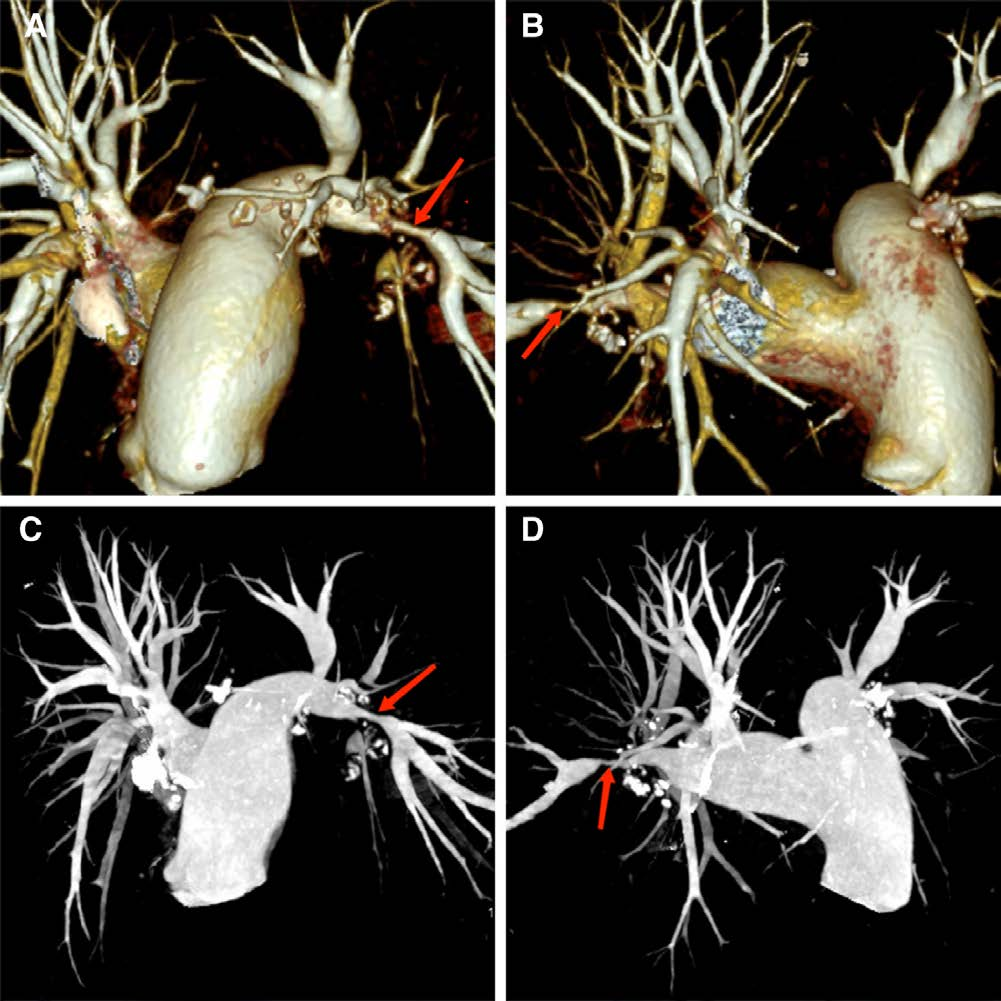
Pulmonary artery CTA findings upon admission. (A and C) Compression and narrowing of the right pulmonary artery branches. (B and D) Compression and narrowing of the left pulmonary artery branches.
